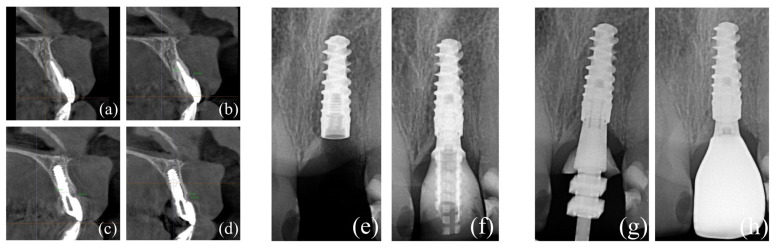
Corresponding radiographic images of the clinical case presented in Figure 7. (a) Pre-fracture CBCT image. (b) CBCT image following vertical root fracture. (c) CBCT image after implant placement, fixation of the autogenous bone graft harvested from the maxillary tuberosity, and placement of the provisional restoration. (d) CBCT image obtained 3 months postoperatively. (e) Intraoral radiograph after implant placement. (f) Intraoral radiograph after placement of the provisional restoration. (g) Intraoral radiograph 3 months postoperatively. (h) Intraoral radiograph after delivery of the final restoration. Colored lines are used only as visual reference markers and do not represent additional quantitative measurements.
